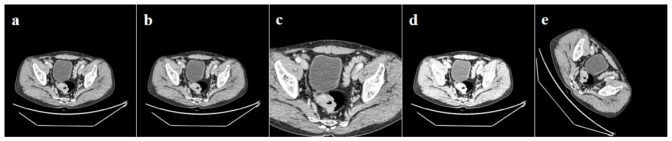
Preprocessing of CT slices. (a), original image; (b), normalization; (c), cropping and resizing; (d), contrast adjustment; (e), rotation.
